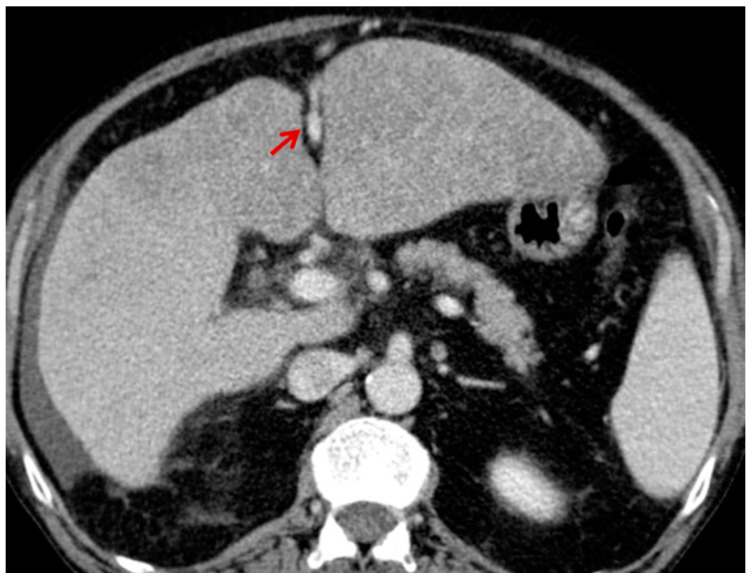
Axial contrast-enhanced CT shows repermeabilized round ligament (red arrow).
